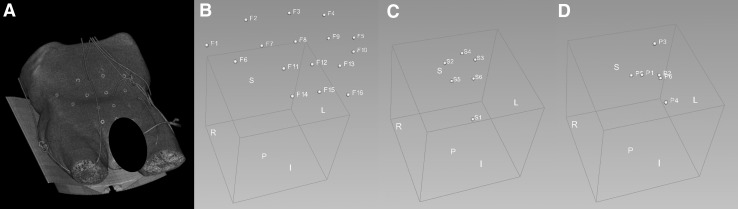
Markers were manually placed in the center of the skin fiducials in each CT-scan by using an image computing platform (3D Slicer) (B). The other markers S1-6 (C) and P1-6 (D) were placed at the anatomical landmarks as depicted in Table 2
